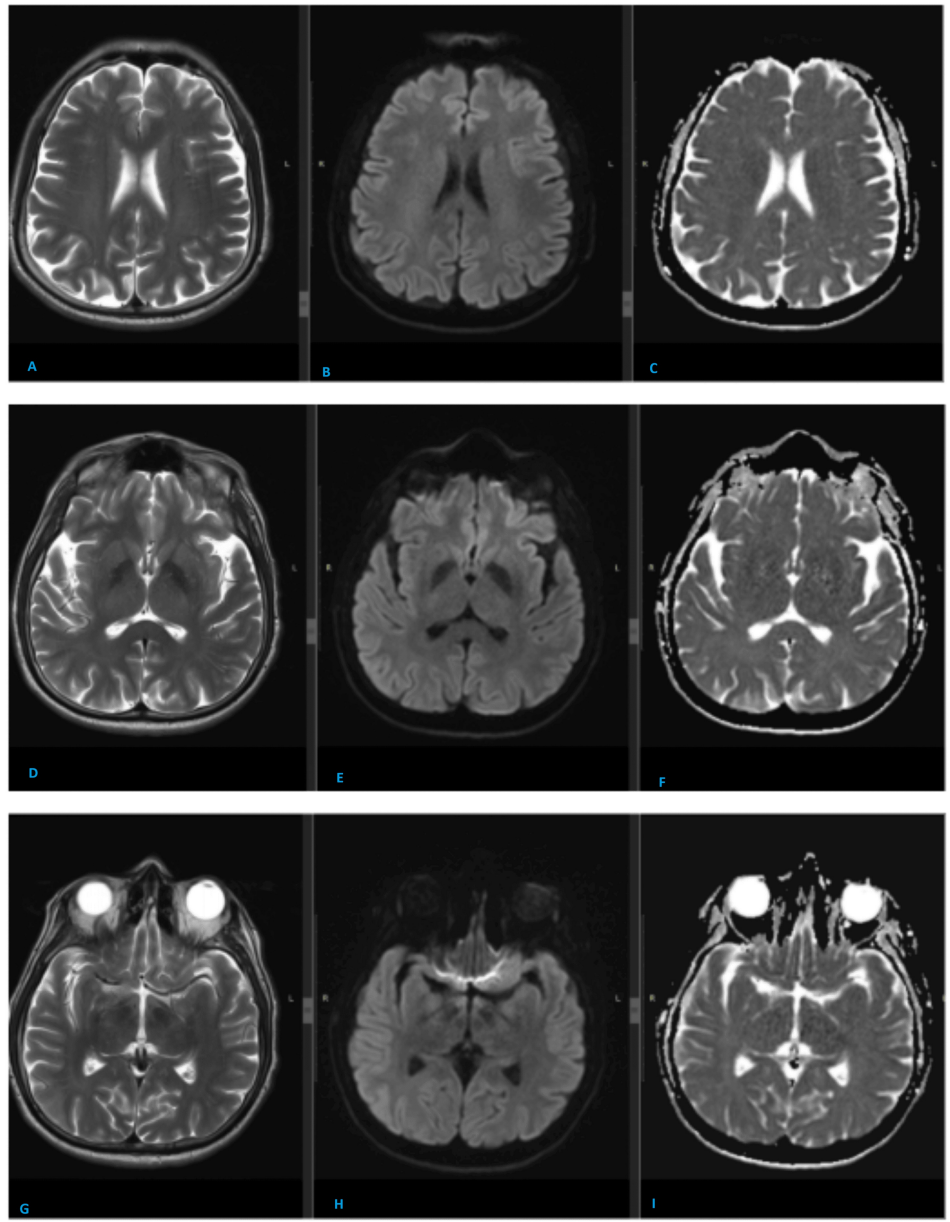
Follow-up MRI brain after approximately six weeks at same levels shows the interval resolution of abnormal signals and diffusion restriction. Follow-up MRI brain shows interval resolution of abnormal T2 high signals in the bilateral frontoparietal white matter (A); trunk and splenium of corpus callosum (D) and left thalamus (G). Also, there is interval resolution of diffusion restriction. Now, there is no abnormal signal on diffusion-weighted imaging (DWI) (B, E, and H) and apparent diffusion coefficient (ADC) sequences (C, F, and I).
